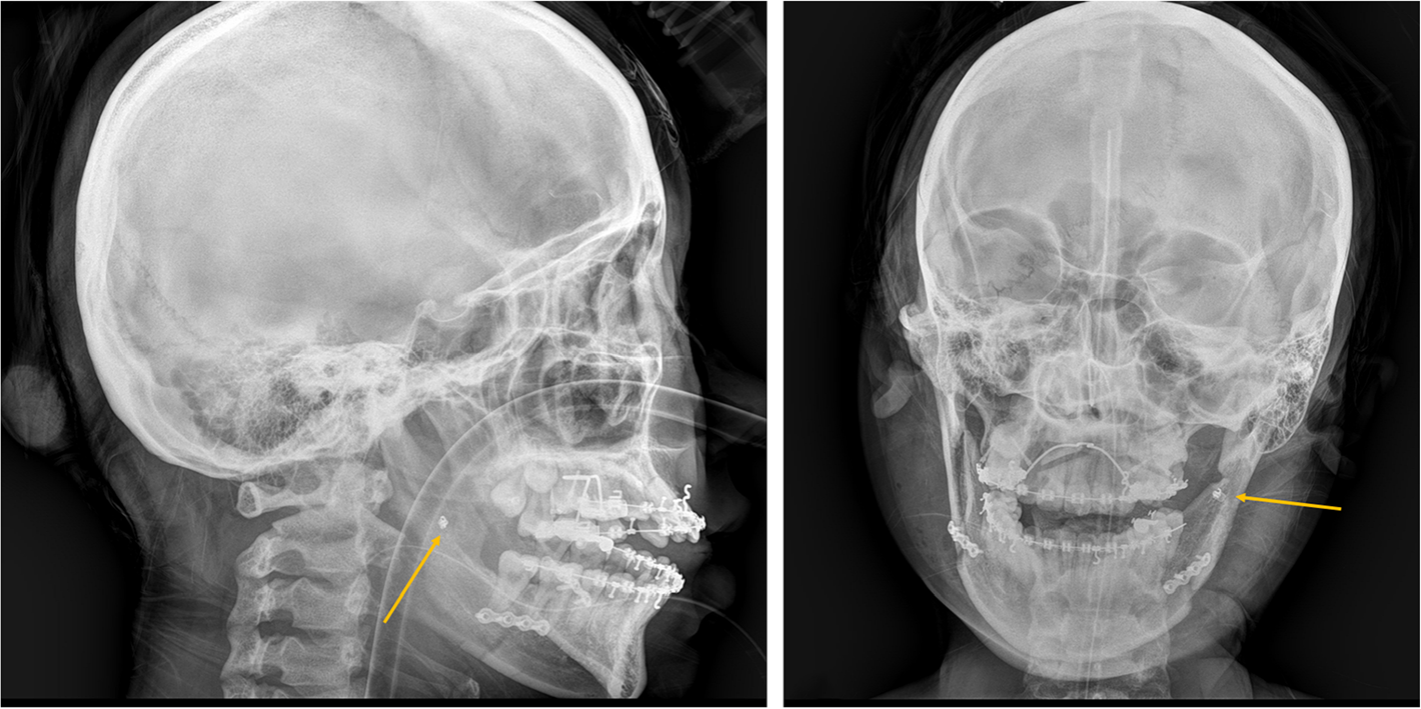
Failed brackets (arrows) are investigated in the operating room using intraoperative radiographic images (a lateral; b frontal image)
